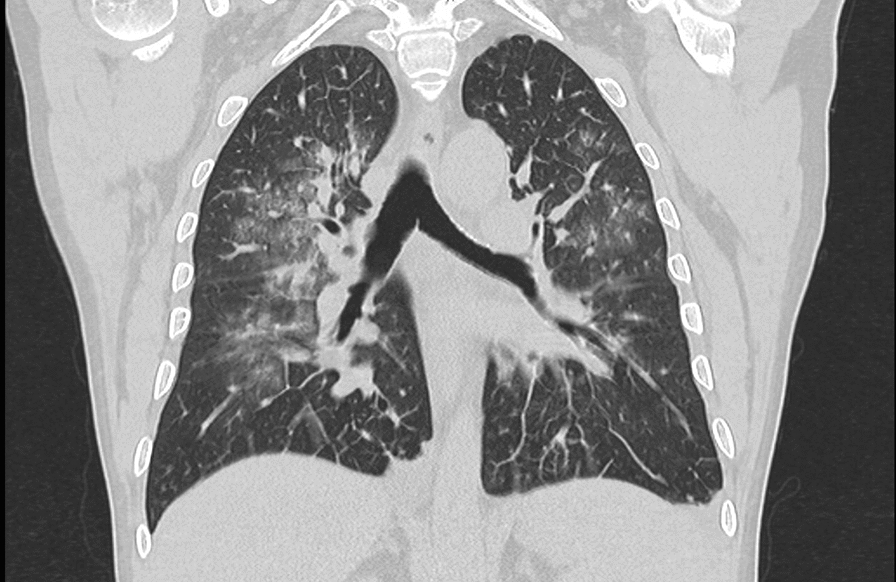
Chest computed tomography during *Plasmodium ovale* malaria showing interstitial bilateral pneumonia with consensual pleural effusion
